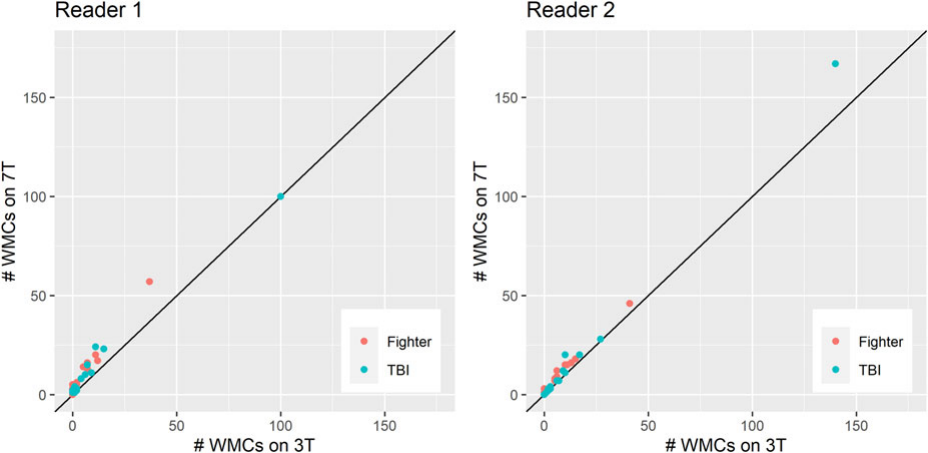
Number of white matter changes (WMCs) detected on 3T MRI versus on 7T MRI among fighters (*n* = 19) and patients with TBI (*n* = 15). MRI, magnetic resonance imaging; T, Tesla; TBI, head trauma.
